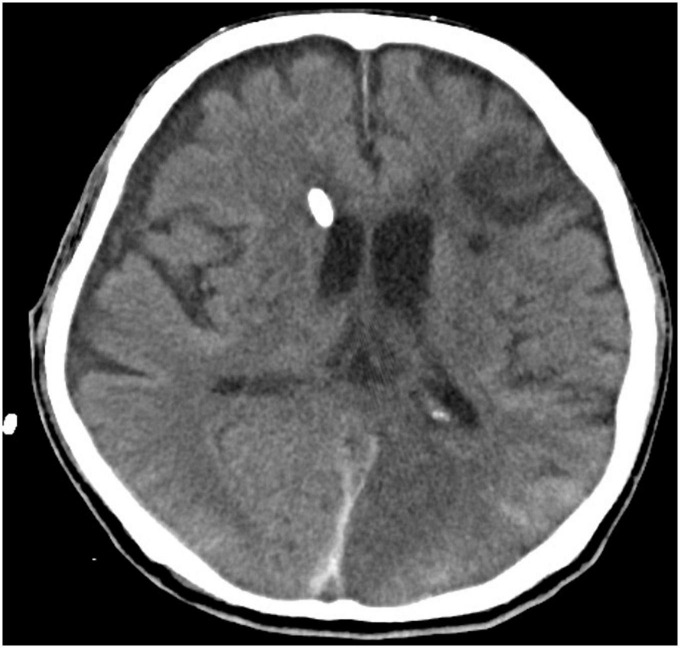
Computed tomography brain with EVD traversing through the right lateral ventricle with reduction of hydrocephalus.
